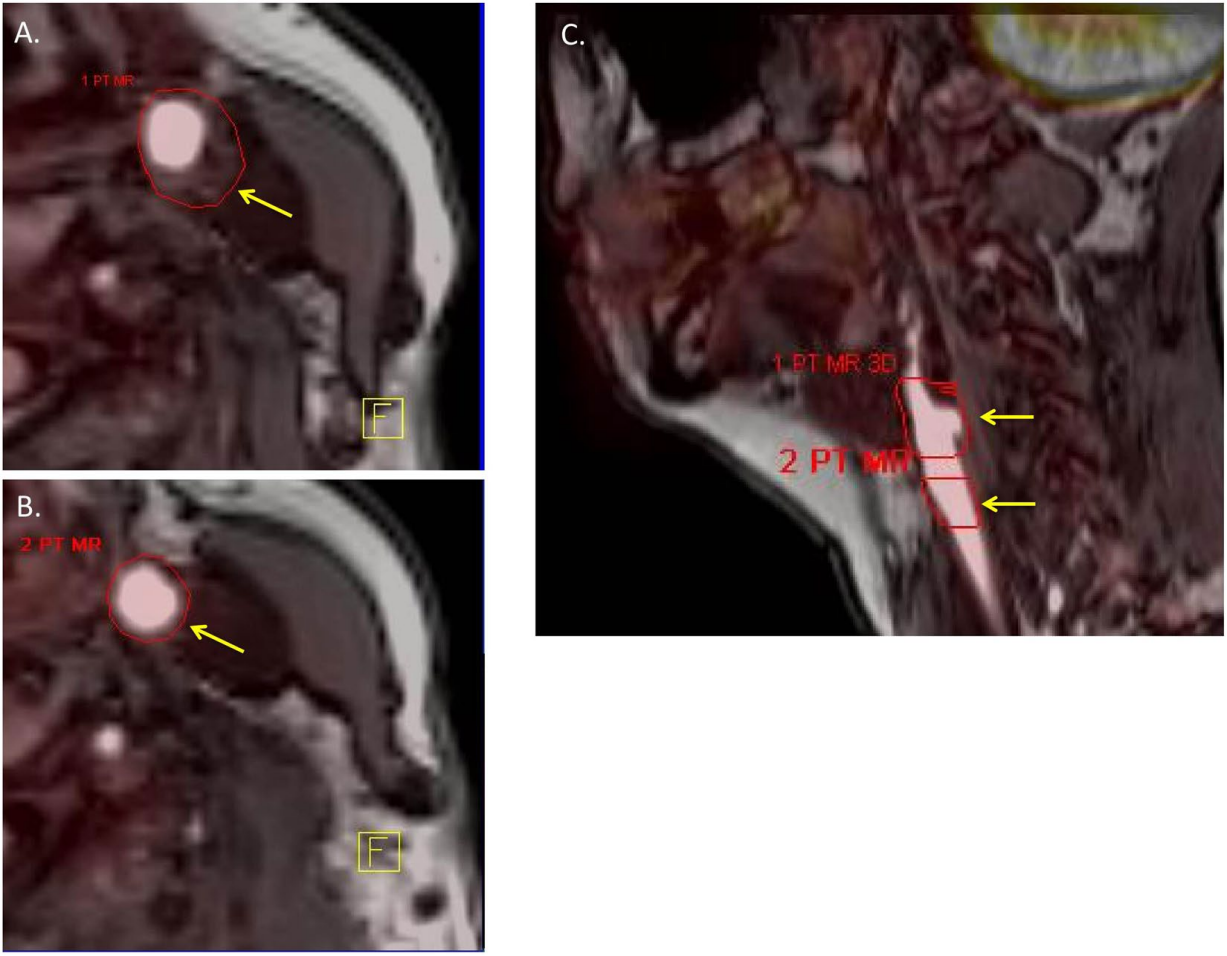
Identification of VOIs on PET-MRI. ROIs were drawn around the borders of plaque on each slice where plaque was seen (an example is seen in the axial image (A), yellow arrow points to VOI border). An equivalent number of ROIs are drawn around vessel wall on slices where no plaques are seen (an example is seen in (B)). An example of the ROIs on a sagittal section can be seen in (C) where each arrow points to the two defined ROIs, the superior one includes the atheromatous wall while the inferior ROI is of non-atheromatous wall.
